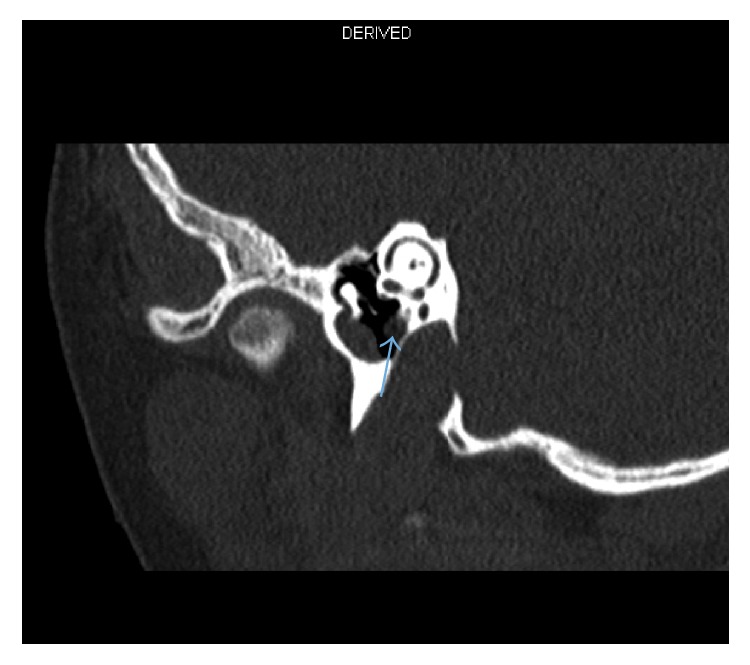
Limited coronal CT scan of right ear revealing a lesion in the area of the second genu of the facial nerve (blue arrow).
